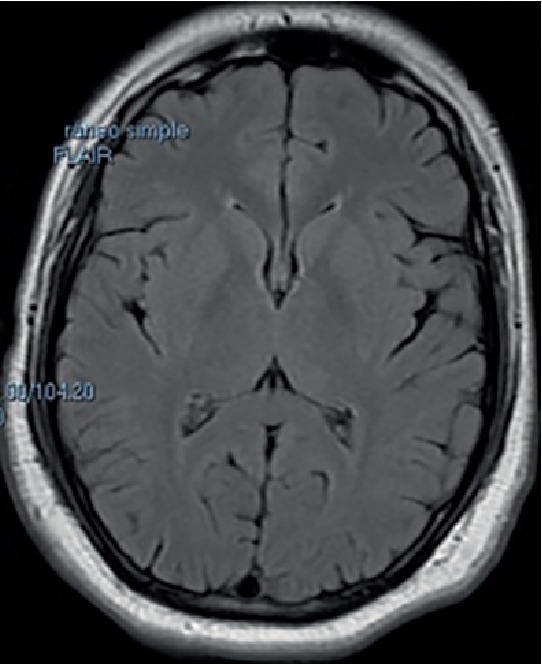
Axial FLAIR showed absence of abnormalities at follow up.
